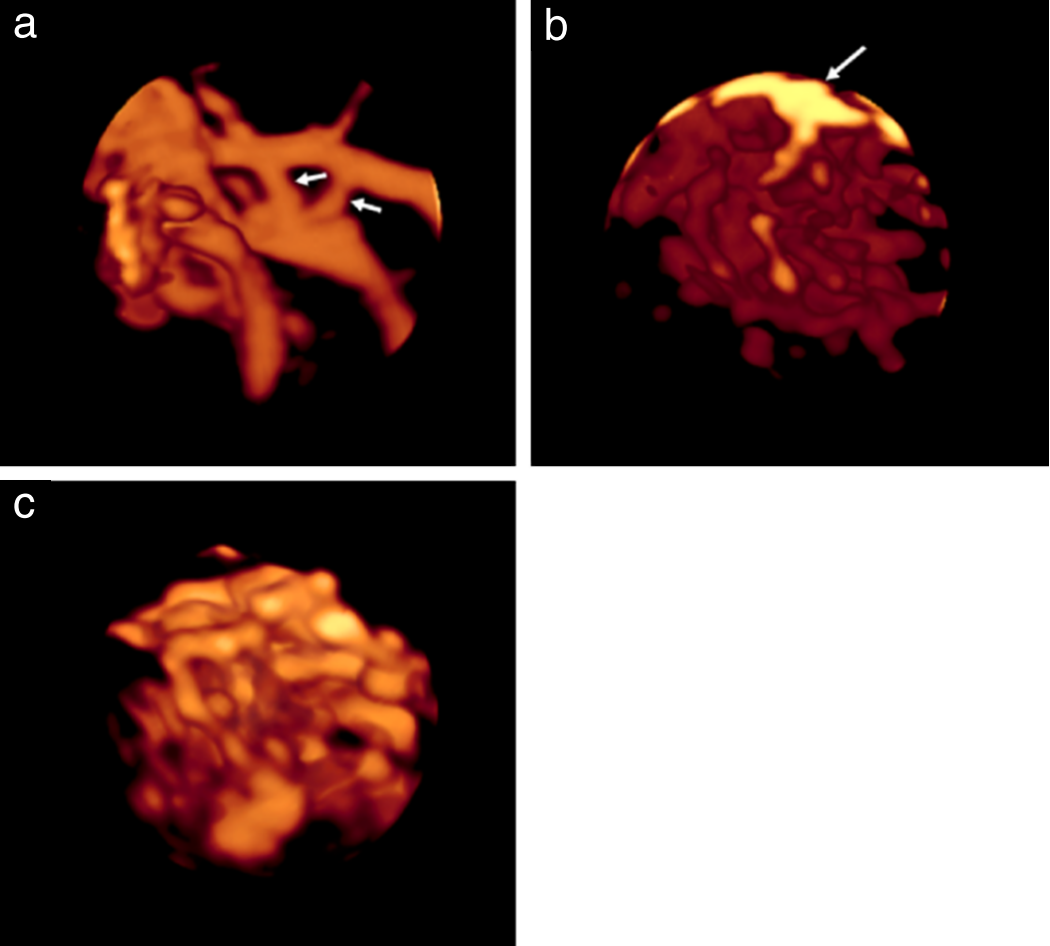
Three‐dimensional power Doppler ultrasound images of vessel tree in 5‐cm^3^ samples of ovarian tumors, showing: (a) bridges (arrows), i.e. straight connections between two nearby vessels, in struma ovarii; (b) branching, tortuous vessels with changes in diameter and color splashes (arrow) in benign ovarian fibroma; (c) branching, tortuous vessels with changes in diameter in ovarian endometrioid carcinoma (color splashes are seen in rotating image of same tumor in Videoclip S7). Corresponding rotating images of vessel trees are shown in Videoclips S5–S7.
